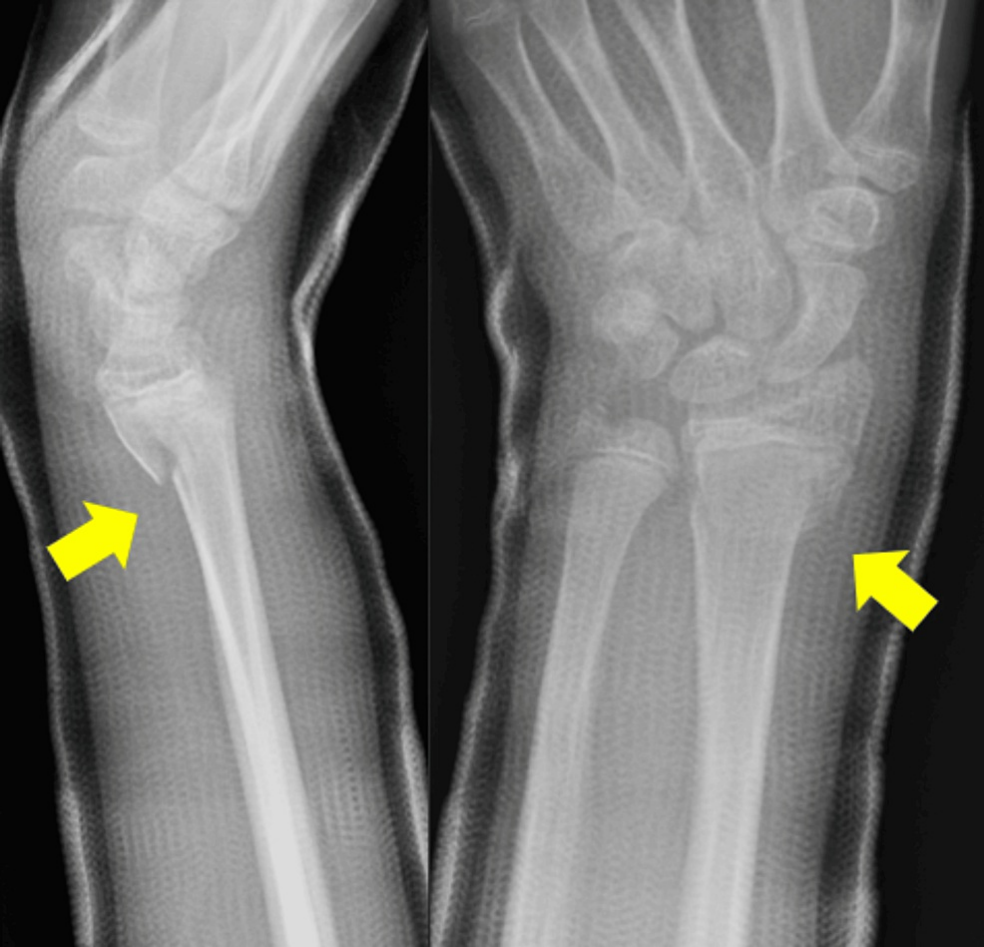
X-rays after manual reduction and immobilization (yellow arrow)
